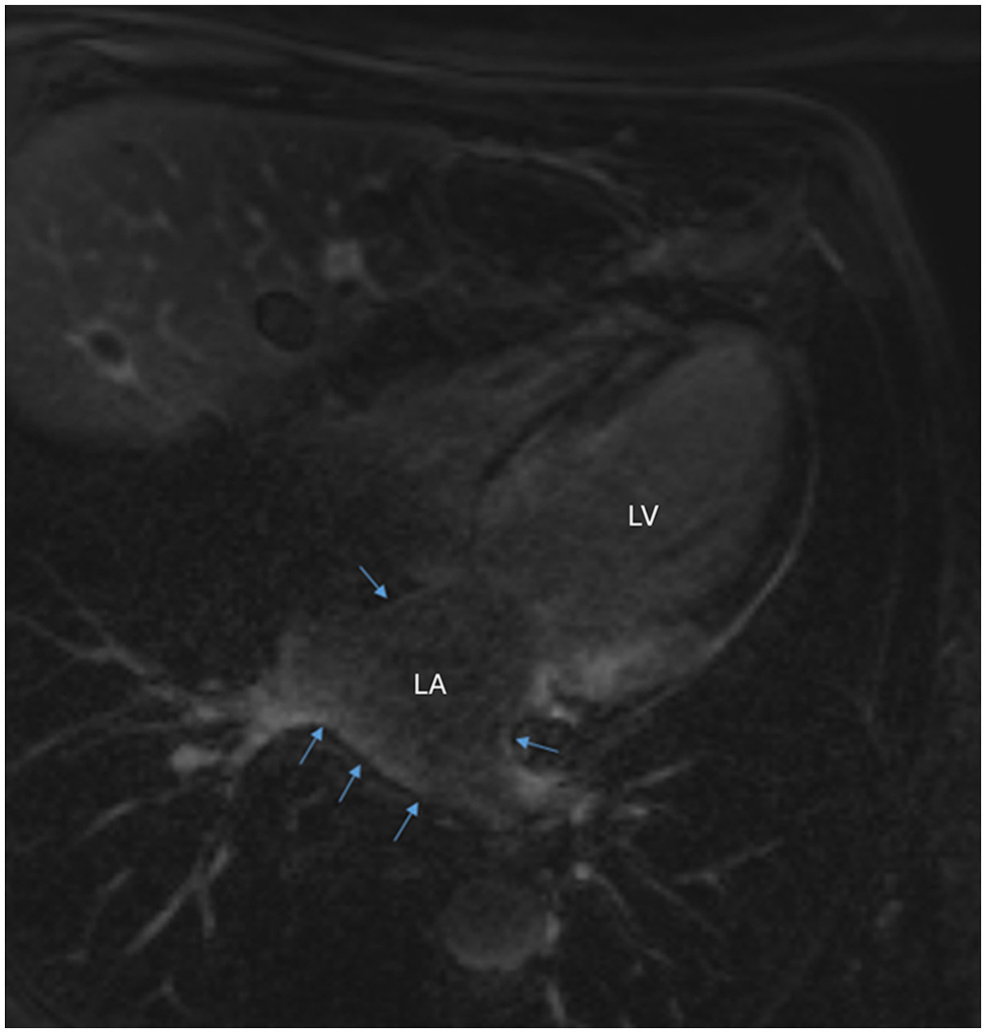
Cardiac magnetic resonance imaging demonstrating diffuse subendocardial late gadolinium enhancement in the left atrium at the level of superior pulmonary veins (blue arrows). LA, left atrium; LV, left ventricle.
